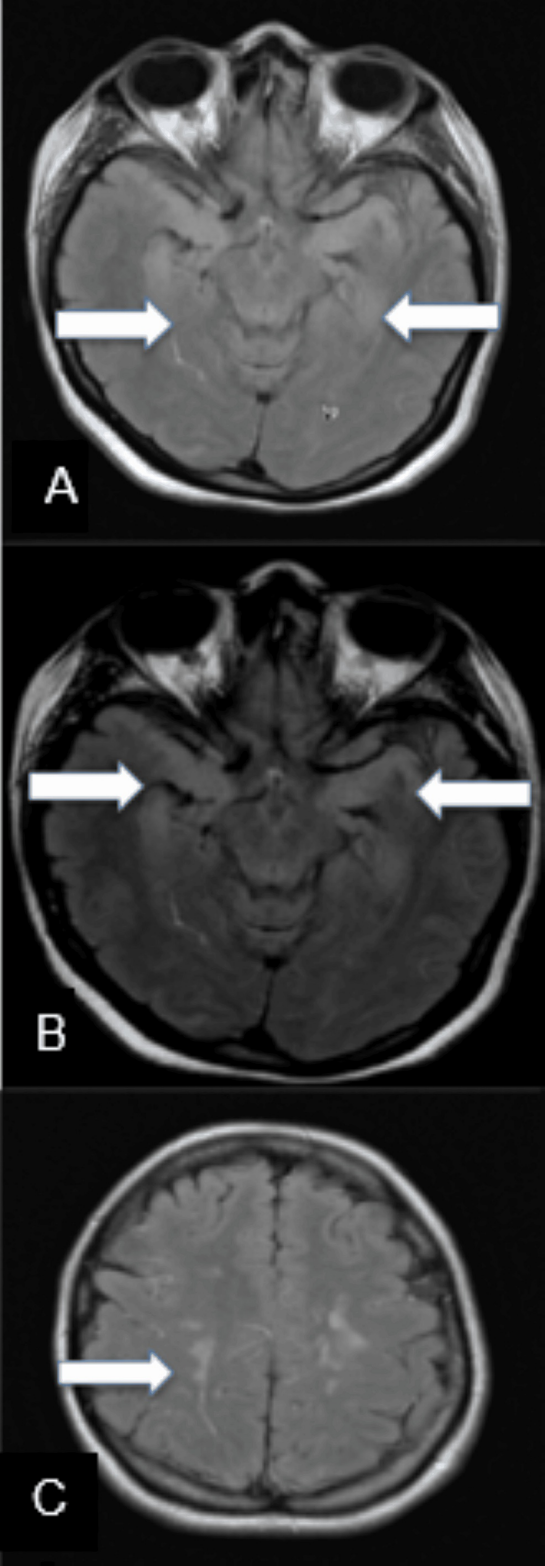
Axial MR FLAIR images of the brain. There is extensive T2/FLAIR hyperintensity of the limbic structures including the hippocampi (A), and temporal amygdalae and para hippocampal gyri (B), as well as the bilateral supratentorial deep white matter (C).
